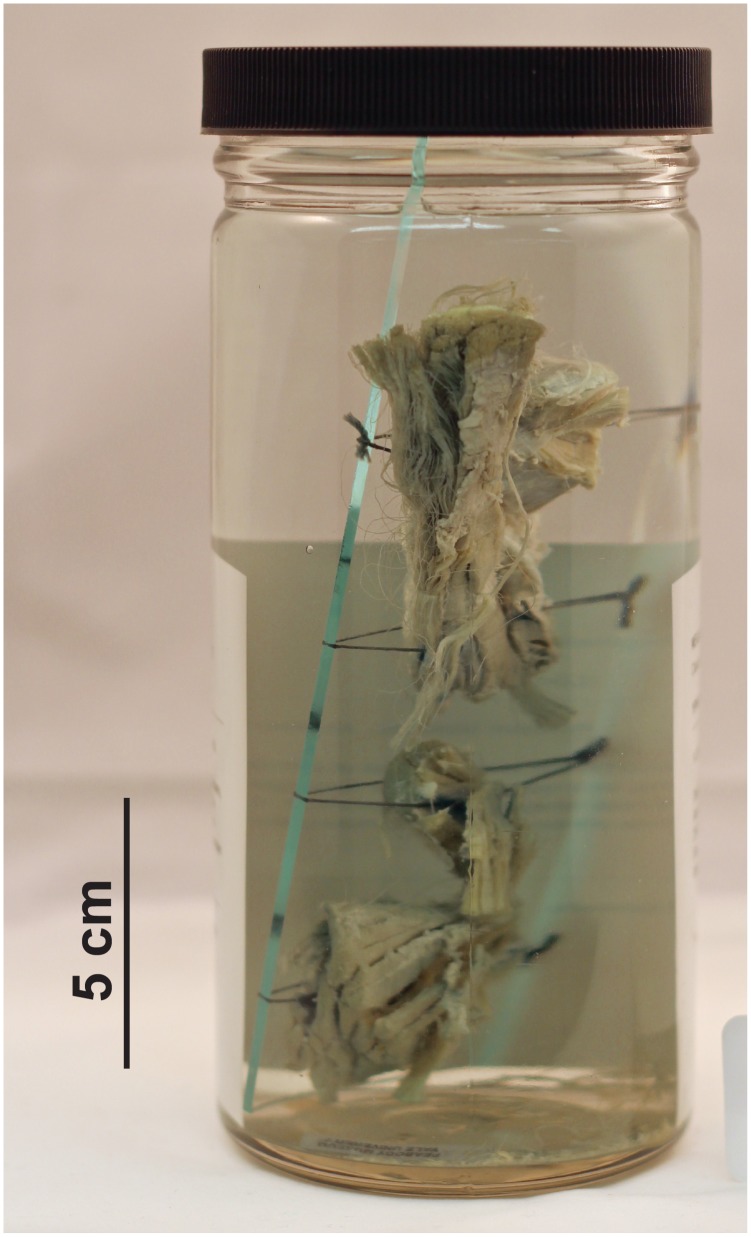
The cooked meat tissue served at the 1951 ECAD. Division of Vertebrate Zoology, YPM HERR 19475. Courtesy of the Peabody Museum of Natural History, Yale University, New Haven, CT, USA.
